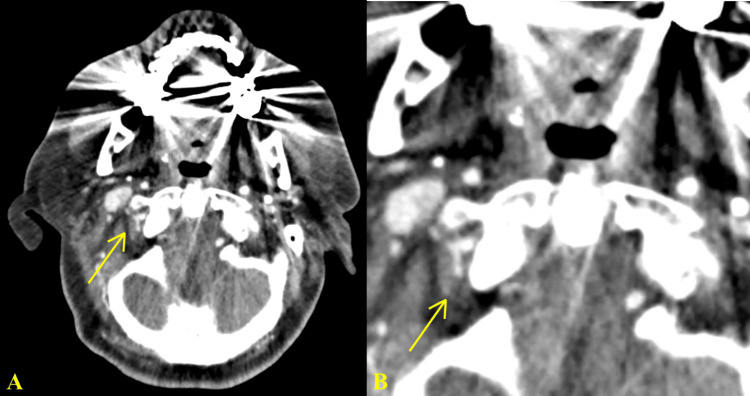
CTA of the neck displaying severe narrowing of the right distal vertebral artery with a string sign at the level of the right C1 loop, most concerning for right distal vertebral artery dissection at this level (A). A magnified image better visualizes the string sign (B). CTA: computed tomography angiogram
